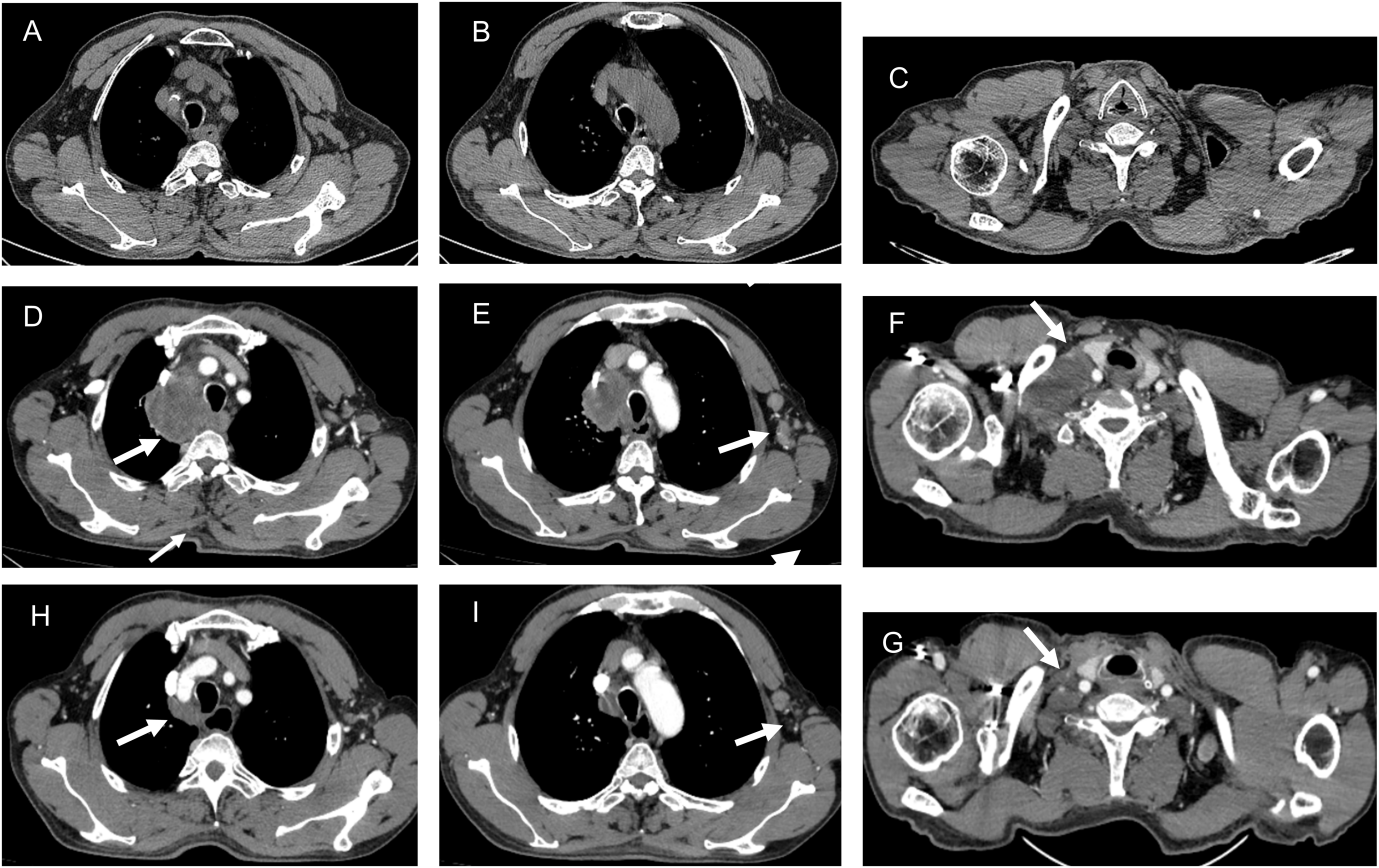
The CT imaging before the first chemotherapy (A-C). The CT imaging following adjuvant chemotherapy with the CAPEOX regimen (D-F). The enlargement of lymph nodes in the mediastinum (D), axilla (E), and right supraclavicular region (F). The CT imaging following nab-paclitaxel and carboplatin combined with camrelizumab (H-G). The regression of lymph nodes in the mediastinum (H), axilla (I), and right supraclavicular region (G).
